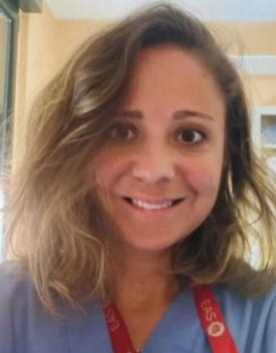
Sara Ruggerini is consultant cardiologist at Ospedale Ceccarini in Riccione. Her field of interest is valvular heart diseases; she mainly works in the echocardiographic laboratory. She is a member of several scientific societies as Italian Society of Cardiology (SIC) and European Society of Cardiology (ESC).
